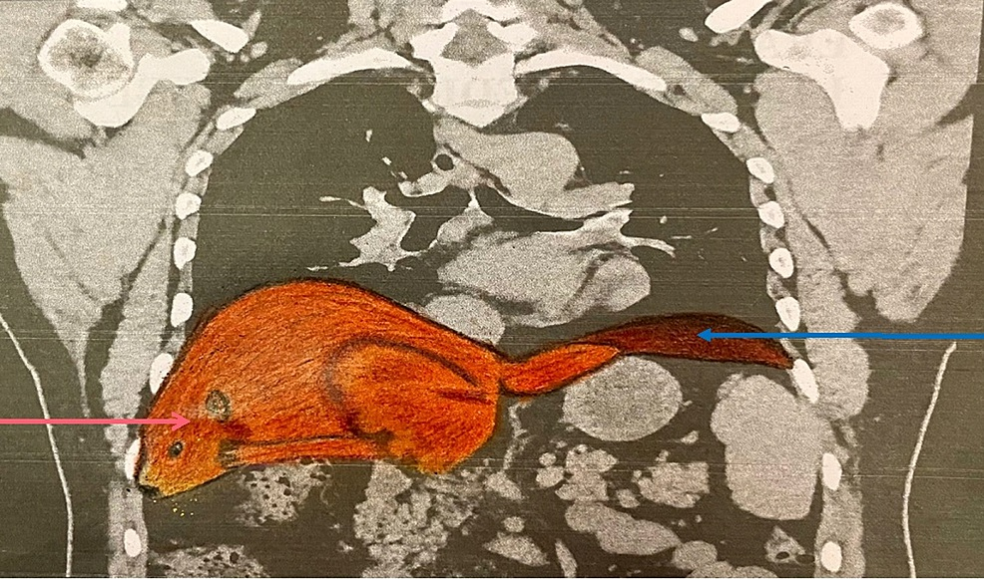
CT demonstrating elongated left lobe of liver mimicking a beaver's tail (blue arrow) with normal right lobe of liver (red arrow).
